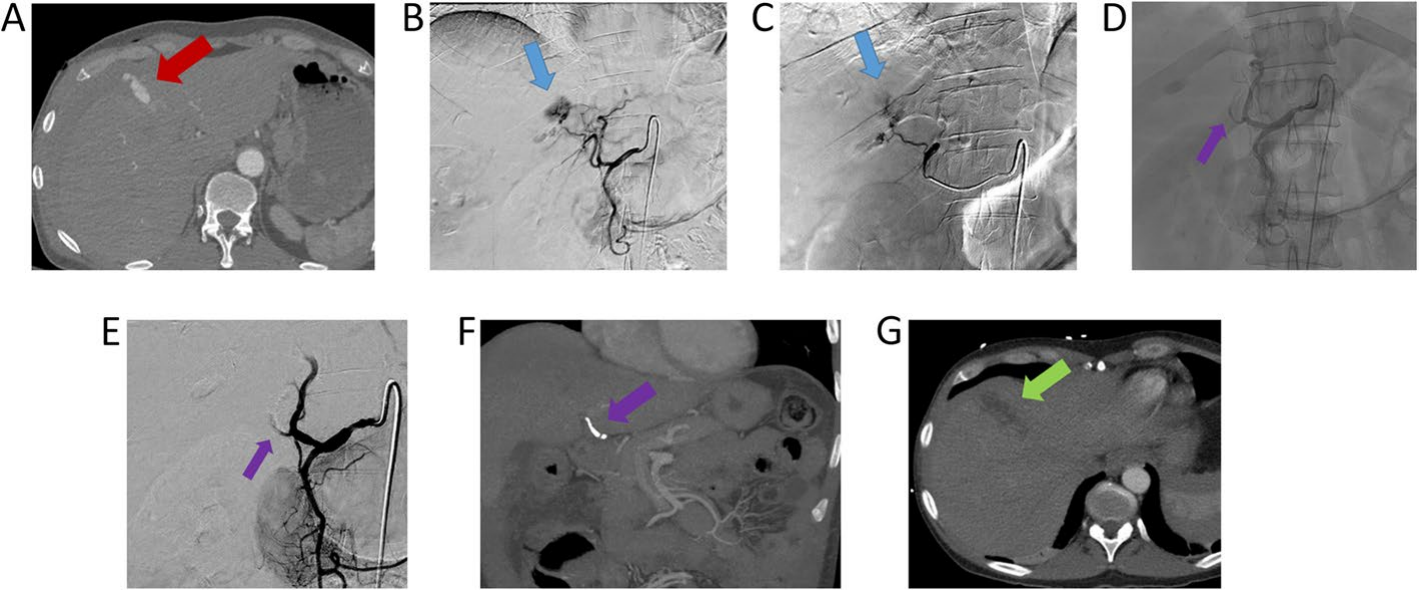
A Computed tomography angiogram demonstrated segment 4 contrast extravasation (red arrow). B and C Celiac and proper hepatic artery angiograms confirmed contrast blush from the segment 4 hepatic artery (blue arrow). D and E Postembolization angiogram showed resolution of segment 4 blush. Purple arrow: Obsidio embolic (D unsubstracted image; E subtracted angiogram). F and G Follow-up computed tomography demonstrated absence of active hemorrhage within the embolization bed (green arrow). Purple Arrow: Obsidio embolic
